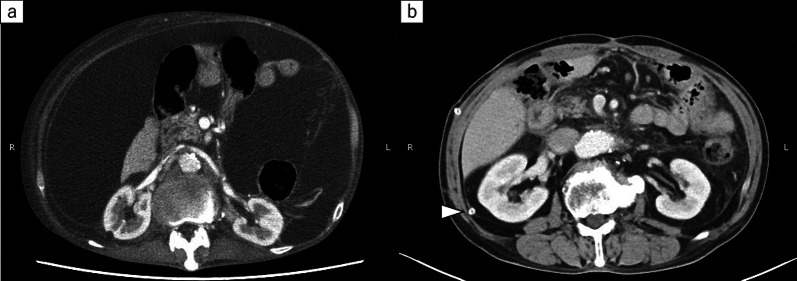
a Abdominal CT showed a large volume of ascites before peritoneovenous shunt. b Abdominal CT showed no ascites at a follow-up examination in the outpatient clinic. Arrow; the peritoneal limb of the PVS
